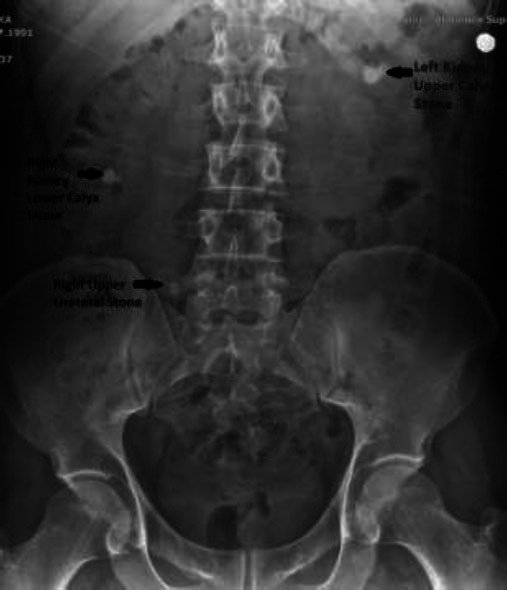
Preoperative KUB radiography of patient 2.
